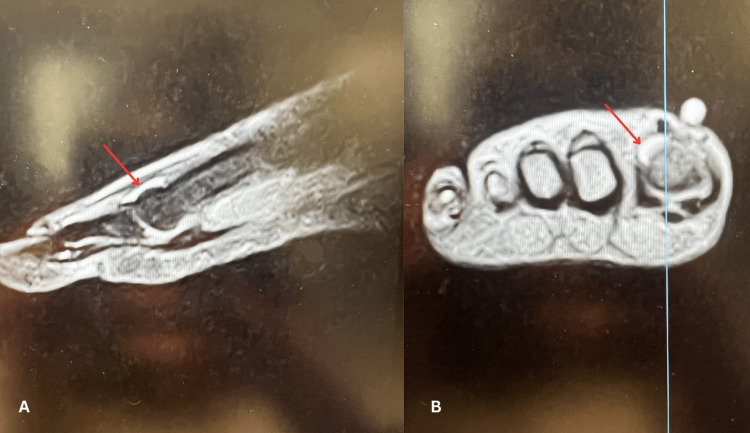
Static MRI sagittal (A) and coronal (B) images demonstrating tenosynovitis of the EHL tendon Tenosynovitis is indicated by the red arrows above. Plane of section in coronal image is shown by the light blue vertical line. EHL: Extensor hallucis longus
